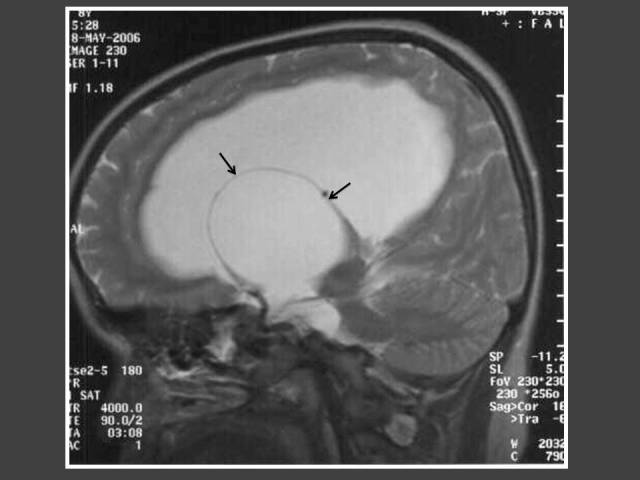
Eight year-old girl who presented with bilateral breast enlargement. T2WI sagittal MRI of the brain shows suprasellar arachnoid cyst (arrows) with hydrocephalus.
